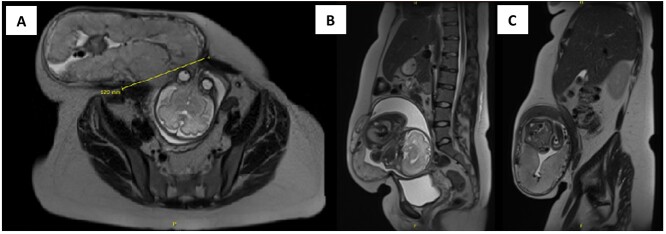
Axial (A) and sagittal (B and C) view of MRI of the abdomen and pelvis showing herniation of the gravid uterus into the right anterior abdominal wall with the neck of defect measuring 12 × 12 cm.
